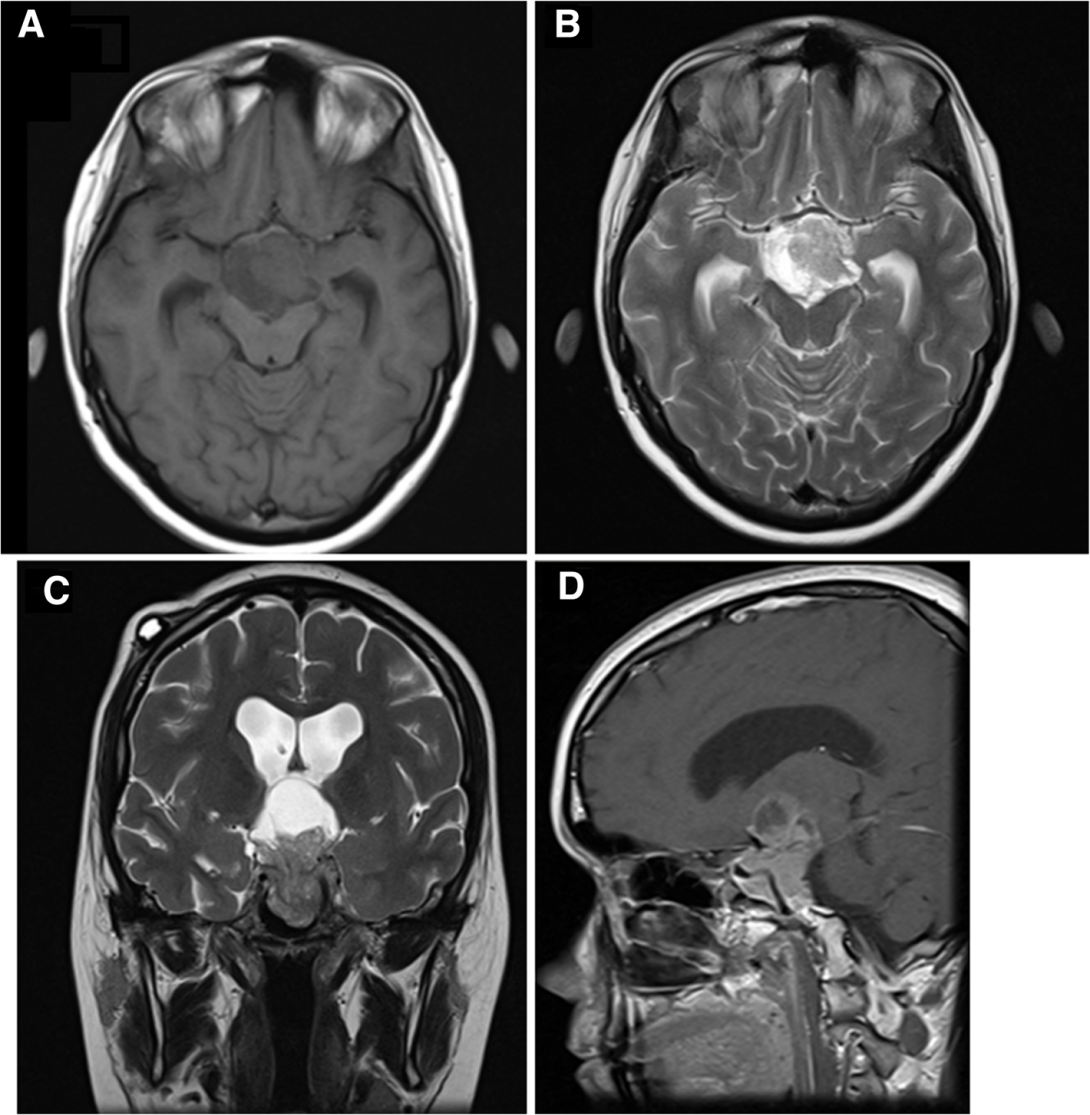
First pituitary magnetic resonance imaging with a giant prolactinoma. Axial T1 (a), axial T2 (b), coronal T2 (c) and sagittal T2-weighted fluid-attenuated inversion recovery images (d)
